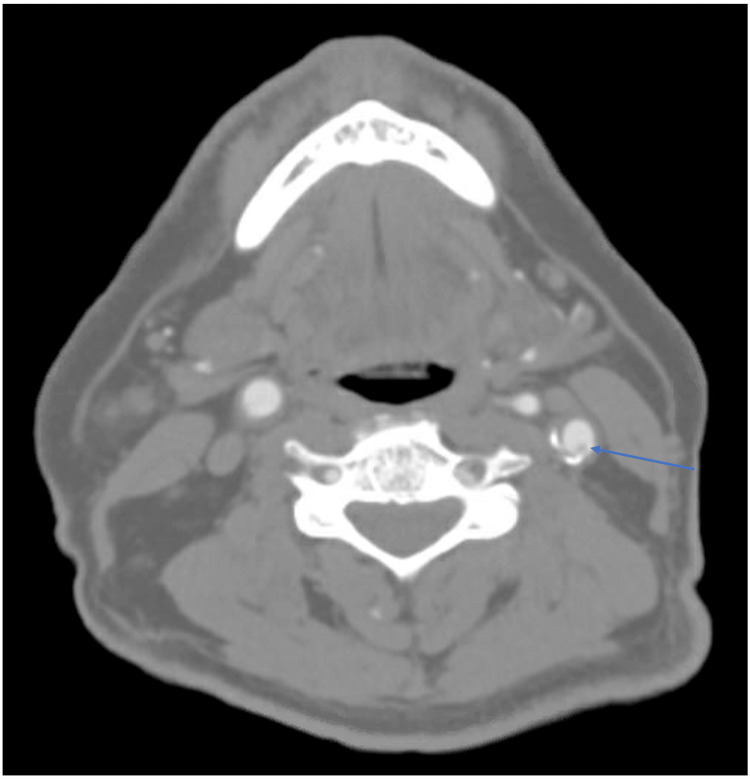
CTA of the head and neck vessels (axial view) demonstrating mixed calcified and soft plaque in the proximal left internal carotid artery protruding from the posterior wall and extending more centrally with no gross hemodynamically significant stenoses (arrow). CTA: CT angiography
